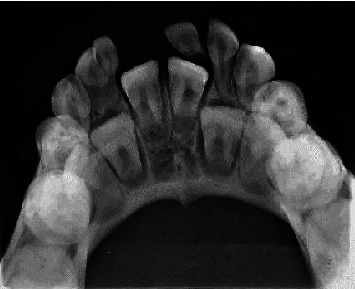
Anterior occlusal radiograph of the mandibular arch showing a type-4 morphology and an incomplete fusion of tooth 72 with a supernumerary tooth.
